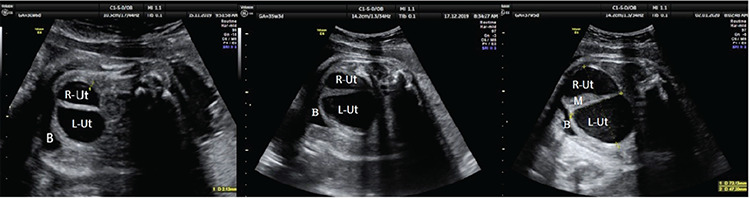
Ultrasound images show the evolution of cloaca accompanied uterus didelphys from 30, 35 and 37 weeks of gestation respectively R-Ut: The right uterine cavity, L-Ut: The left uterine cavity, B: Bladder, M: Meconium
